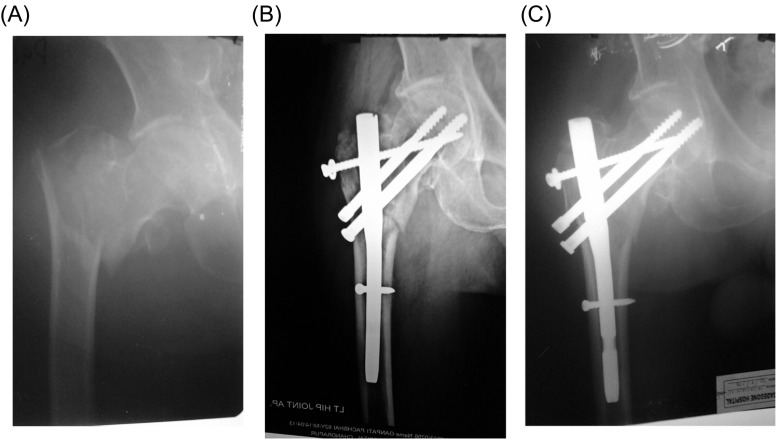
A 75-year-old male. (A) The initial preoperative radiographs. (B) Radiograph six weeks postoperatively with additional screw with washer. (C) Anteroposterior radiograph at 10 months postoperatively demonstrating the fracture healing.
